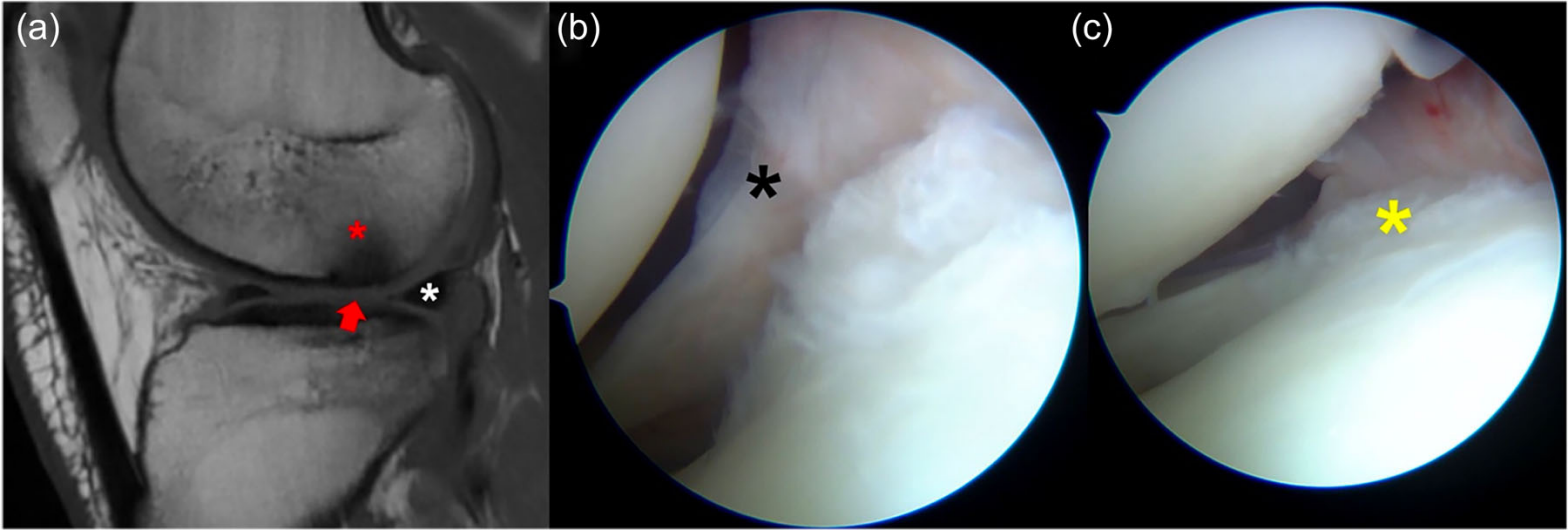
Right knee of an 18‐year‐old patient with a chronic ACL tear. The sagittal MRI of the lateral compartment shows the presence of subchondral bone oedema (red asterisk) and cartilage thinning (red arrow) at the lateral femoral condyle, with an intact lateral meniscus (white asterisk) (a). At the time of arthroscopy, the posterior root (black asterisk) was torn from the tibial insertion and floating (b). The root (yellow asterisk) was repaired with an all‐inside suture of the remnant (c). ACL, anterior cruciate ligament; MRI, magnetic resonance imaging.
